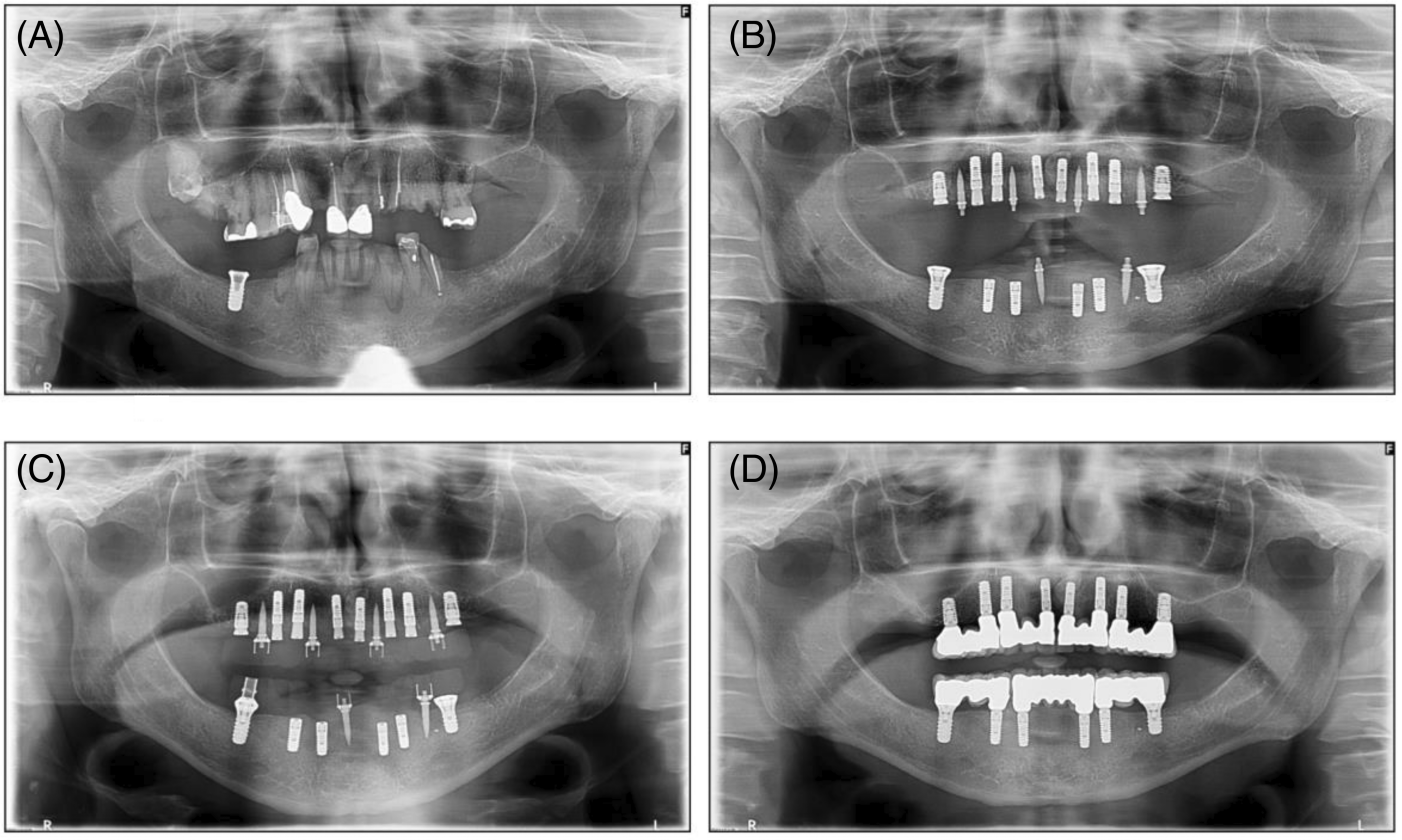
(A) Initial panoramic radiograph (pretreatment); (B) Position of regular implants and TIs postsurgery in the maxilla and mandible; (C) Loading of provisional prostheses onto maxillary TIs and onto mandibular TIs and one regular implant that was already in the jaw pretreatment; (D) Final fixed prostheses in the maxilla and mandible supported by regular implants.
